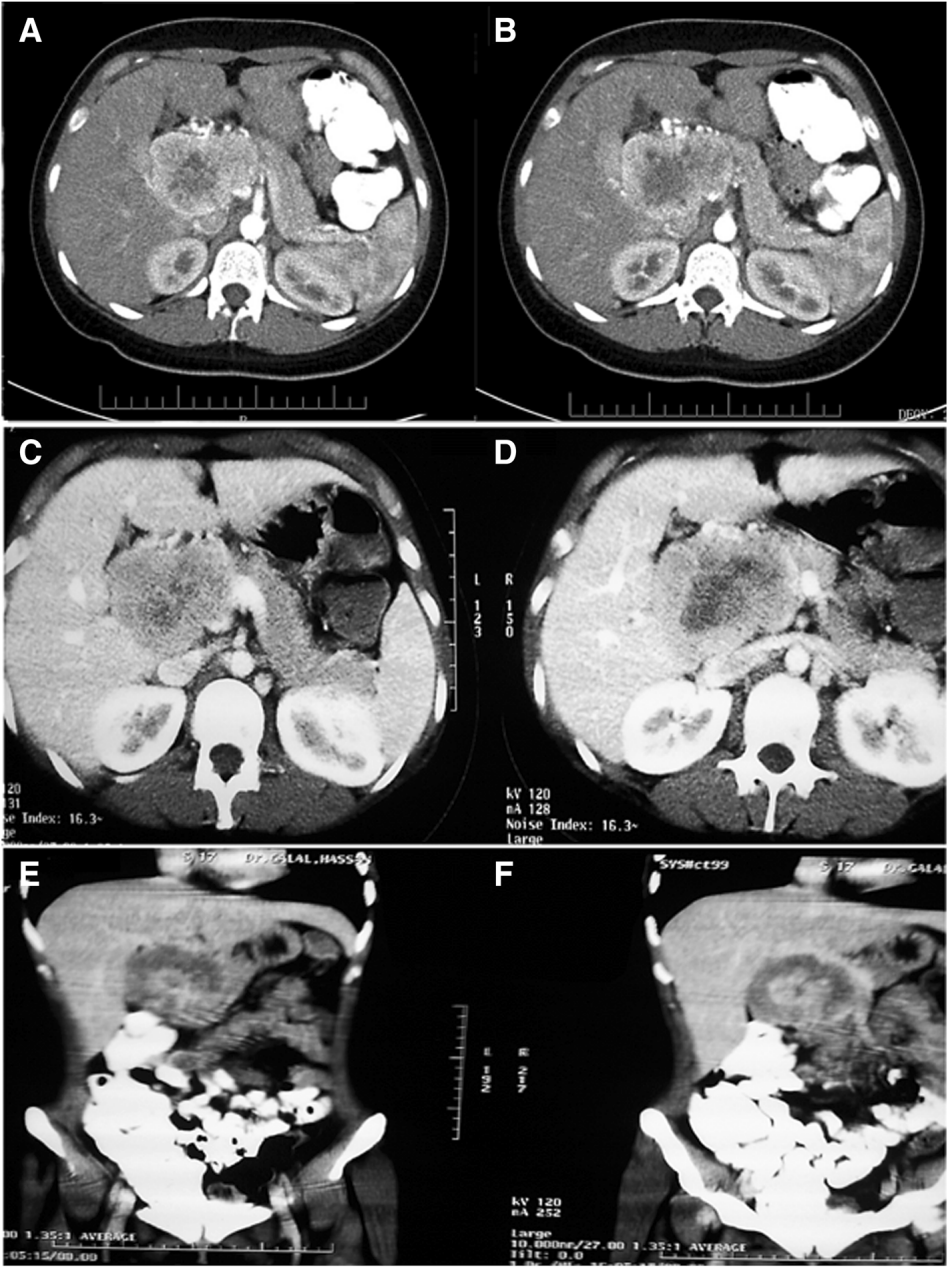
A computed tomography scan demonstrating a large, well defined pancreatic mass. A-D shows the mass in the pancreatic head and ancinate process with marked enhancement in the arterial phase. E-F: The lesion was anterior to the aorta and inferior vena cava, anteromedial to the right kidney, abutting the fundus of the gall bladder, and reaching the level of porta hepatis.
